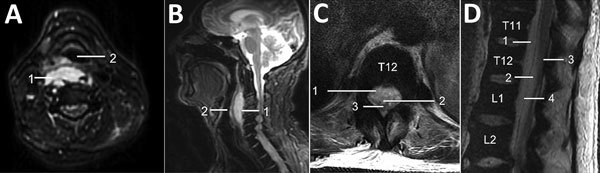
Magnetic resonance imaging of a 60-year-old immunocompetent man with methicillin-resistant *Staphylococcus aureus* clonal complex 398 infection. A, B) Axial (A) and sagittal (B) T2-weighted fat-suppressed sequences of the cervical spine demonstrate a retropharyngeal abscess (1) that moderately anteriorly displaces and mildly effaces the hypopharynx (2). C, D) Axial (C) and sagittal (D) T2-weighted MRI sequences of the thoracolumbar spine (T11–L2 vertebra levels labeled) demonstrate a large ventral, combined epidural (1) and subdural (2) spinal collection that displaces the conus medullaris (3) dorsally. Note the dura mater (4) on the sagittal sequence, which delineates the theca and separates the epidural and subdural spaces.
